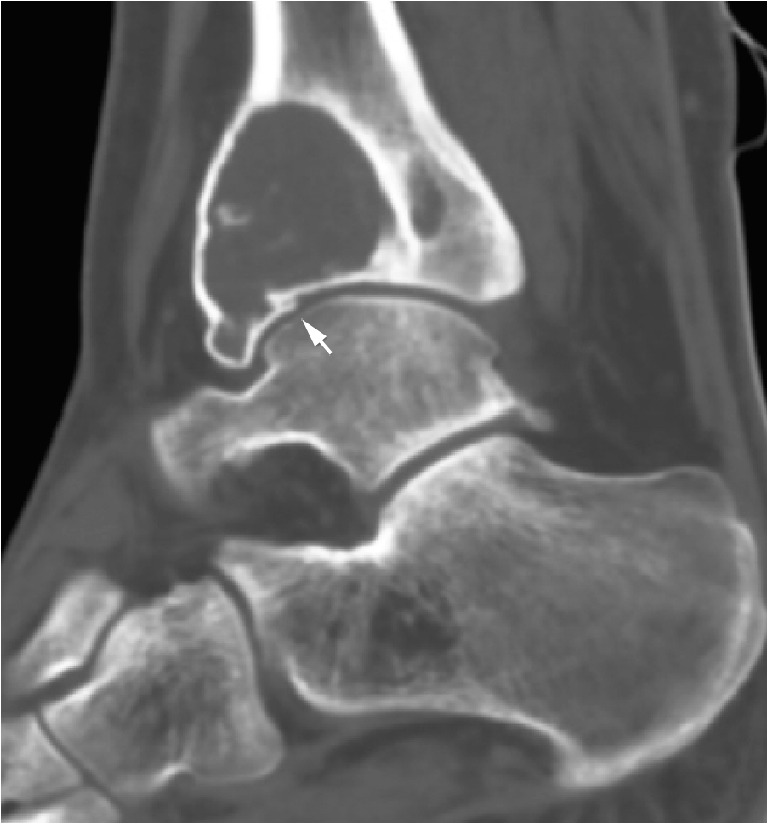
A 20-year-old male with known GCTB of the distal tibial epiphysis. CT sagittal reformat after 8 weeks on denosumab treatment. The GCTB is seen as a mildly expansile, eccentric lytic lesion with the development of marginal sclerosis and irregularity of the tibial articular surface anteriorly (arrow) while on denosumab
